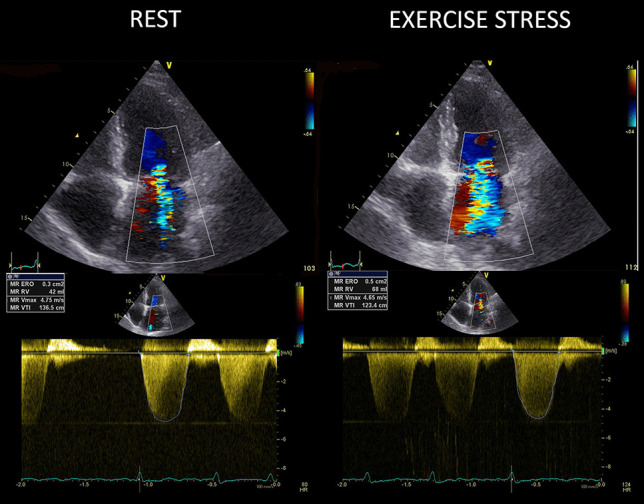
The worsening MR pattern during stress. Apical 4-chamber view showing color-flow Doppler (upper panels) and continuous wave Doppler (lower panels) at rest (left panels) and at peak exercise (right panels) in a patient with a large exercise-induced increase in mitral regurgitation. ERO, effective regurgitant orifice; RVol, regurgitant volume. ERO increases from 0.3 cm^2^ at rest (moderate) to 0.5 cm^2^ at peak stress (severe). Courtesy of Dr. Angela Zagatina, Saint Petersburg, Russia, part of the Stress Echo 2030 study group
